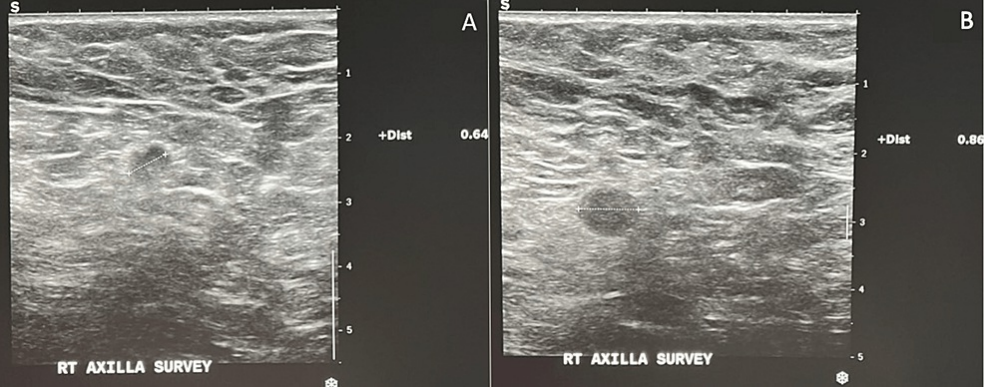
Ultrasound of the right axilla. A. A 0.64 cm lymph node. B. A 0.86 cm lymph node. Both nodes have an irregular shape and loss of their fatty hilum.
